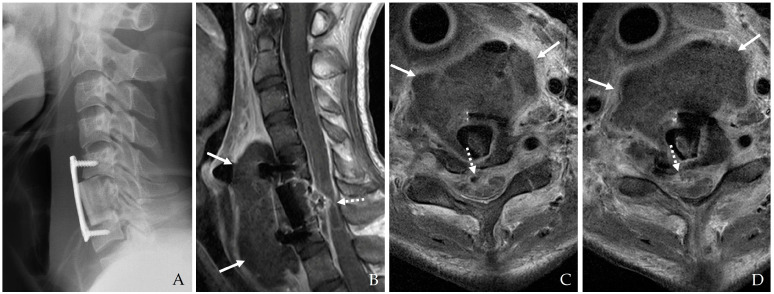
The patient underwent C5-6-7 anterior cervical discectomy and fusion (A). At postoperative 2 months, postoperative infection occurred, and magnetic resonance images showed retropharyngeal (white arrows) and epidural (dotted white arrows) (B–D).
